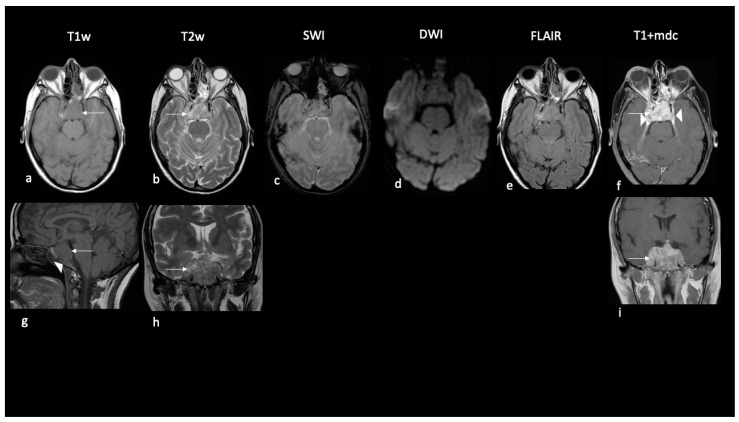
Imaging findings of sellar ATRT in an adult. Ax T1w (a,g); Ax T2w (b,h); SWI (c); Ax DWI (d); Ax FLAIR (e); Ax T1w+mdc (f,i). A local aggressive mass centered in the sellar region in an adult of 43 years. The mass is prevalently solid, hypointense in T1w (arrows in (a,g)) and hyperintense in T2w (arrows in (b,h)) to gray matter with marked enhancement (arrow in (f,i)) invading cavernous sinus (arrowheads in (f)) and clivus (arrowhead in (g)). Decreased diffusivity and interstitial edema are absent.
